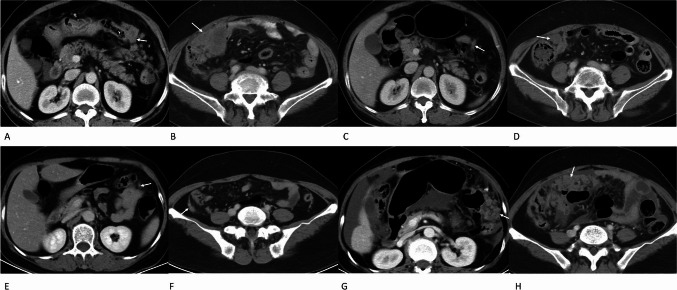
A 61-year-old woman with PM in gastric cancer (A, B). Enhanced CT images before chemotherapy showed confluent PM lesions larger than 5 cm in the left upper region of No. 3 (A) and the right lower region of No. 7 (B), indicating a score of 3, respectively. After first-line chemotherapy, the lesions decreased in No. 3 with a score of 2 (C) and No. 7 with a score of 2 (D). The total CT-PCI score was 18 after chemotherapy compared with the PCI score of 30 in the baseline CT. The OS of this patient was 13 months. A 56-year-old woman with PM in gastric cancer (E–H). Enhanced CT images before chemotherapy showed a PM nodule less than 5 mm in the left upper region of No. 3 with a score of 1 (E) and a 10 mm PM nodule in the right lower region of No. 7 with a score of 2 (F). After first-line chemotherapy, the lesions increased in No. 3 with a score of 3 (G) and No. 7 with a score of 3 (H). The total CT-PCI score increased to 33 after first-line chemotherapy from a PCI score of 21 on baseline CT. The OS of this patient was 6 months
